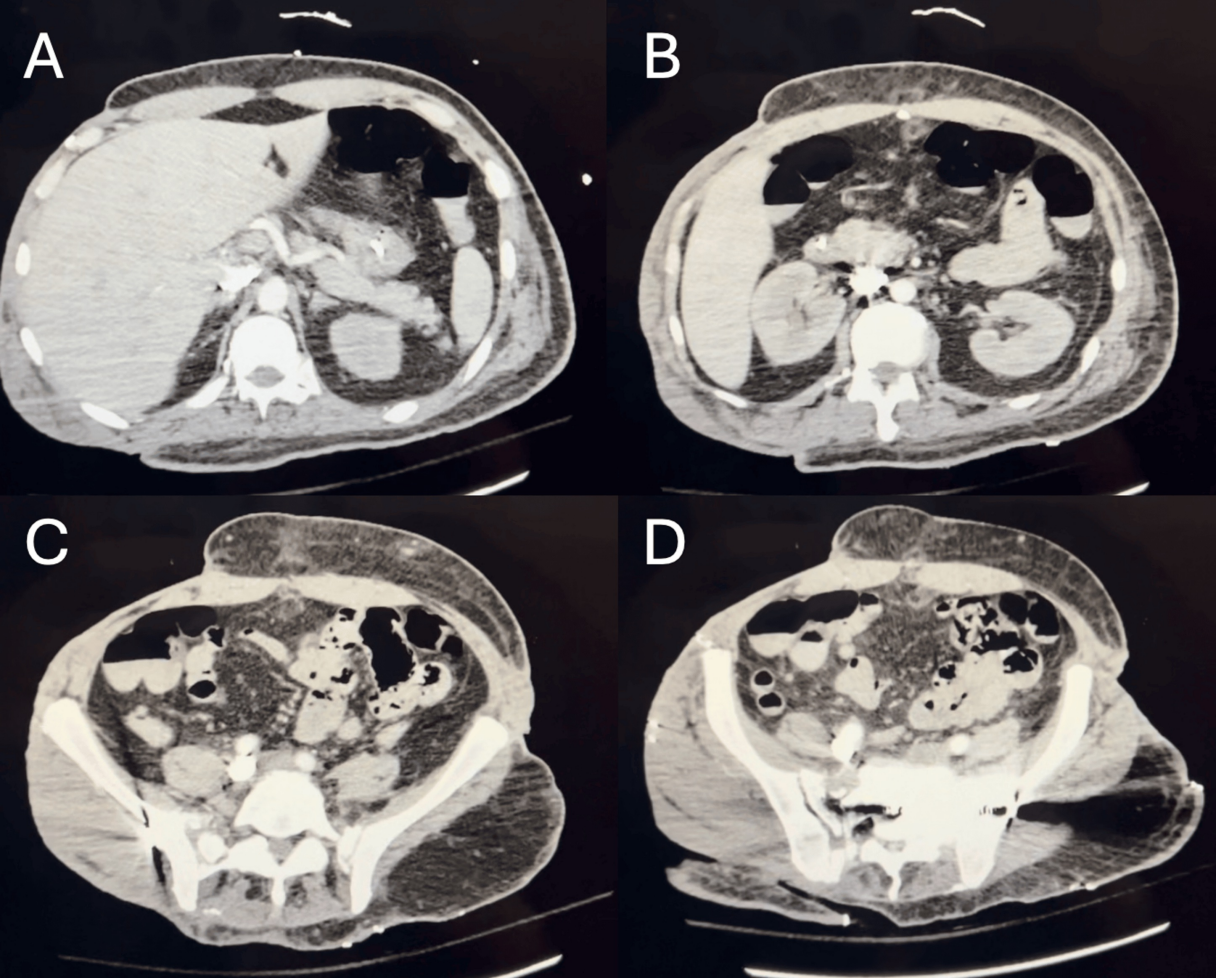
Day 81 computed tomography angiography Imaging of the celiac (A) and superior mesenteric (B) arteries, as well as the gastrointestinal tract (C and D), showed no evidence of active bleeding.
